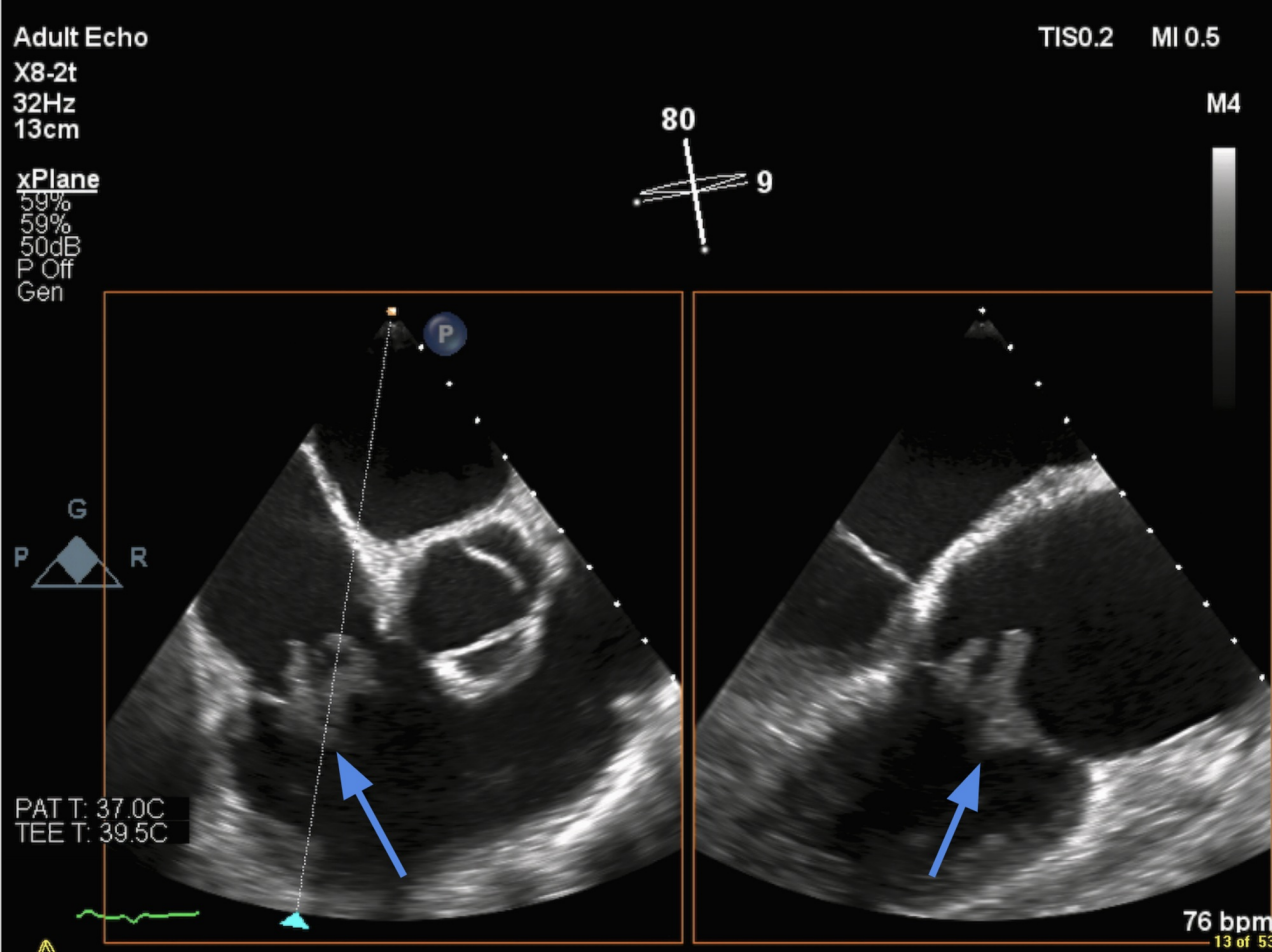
A 2-D echocardiography image of the tricuspid valve showing significant vegetations
